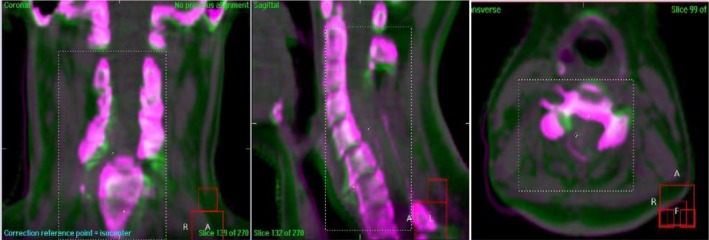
X‐ray volumetric images (pink) overlaps with planned CT images (green) (matching area in dotted box).
